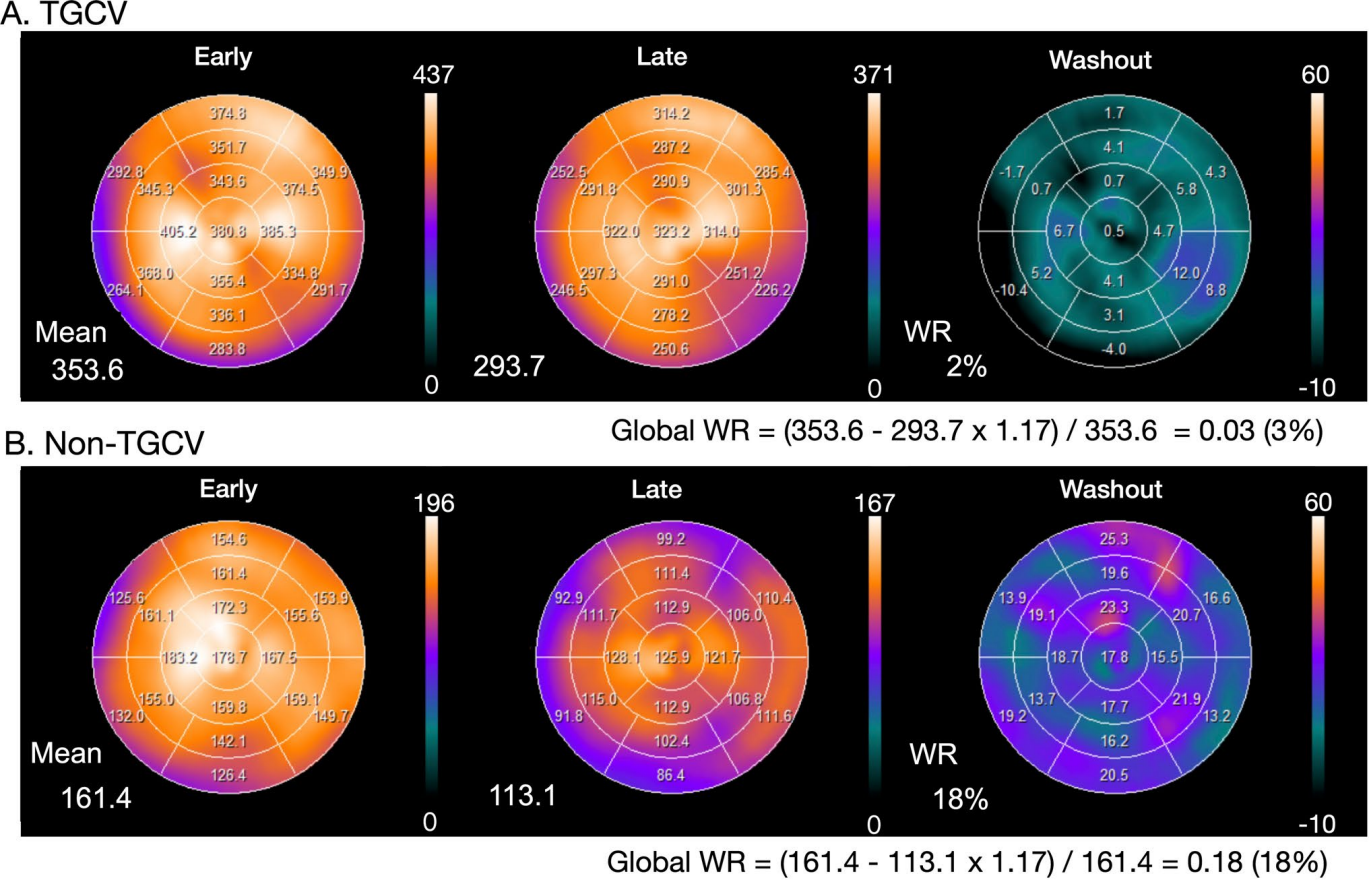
Examples of WRs in SPECT polar maps. A Clinical diagnosis of TGCV with WR 3%. B Coronary artery disease with WR 18%. Late image is shown with color scale in which maximum count is multiplied by a time-decay correction factor of 3 h (1.17). Global WR can be calculated by defining WR (Eq. 1) after decay correction. Compared with average of pixel-based WR (left lower corner of WR polar map, 2%), global WR (3%) was in agreement when regional WR values were homogeneous. However, averaged pixel-based WR might be influenced by misaligned early and late slices or defective regions. *WR* washout rate
